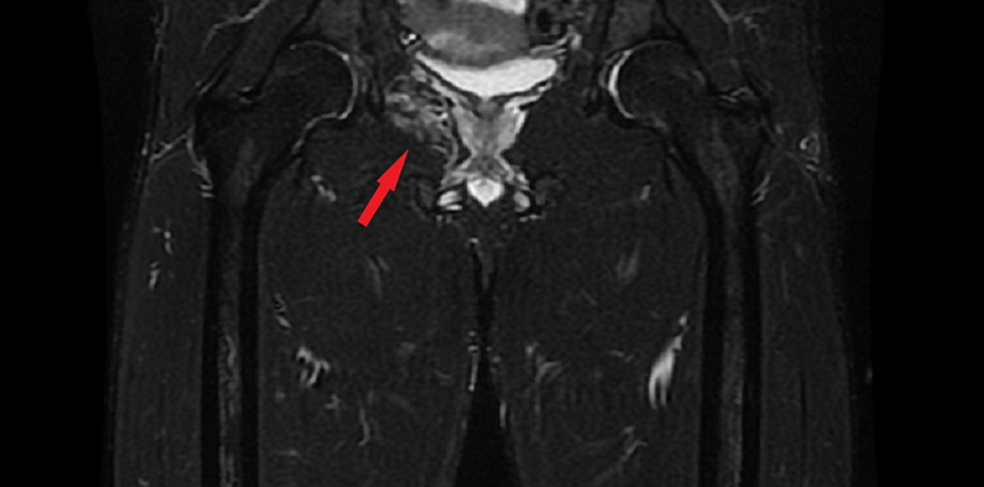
MRI STIR Sequence Coronary View Coronary view of the right hip. Intense signal (Grade II muscle strain) in the obturator internus at the intrapelvic route. The red arrow shows the obturator internus. STIR: Short Tau Inversion Recovery Image
